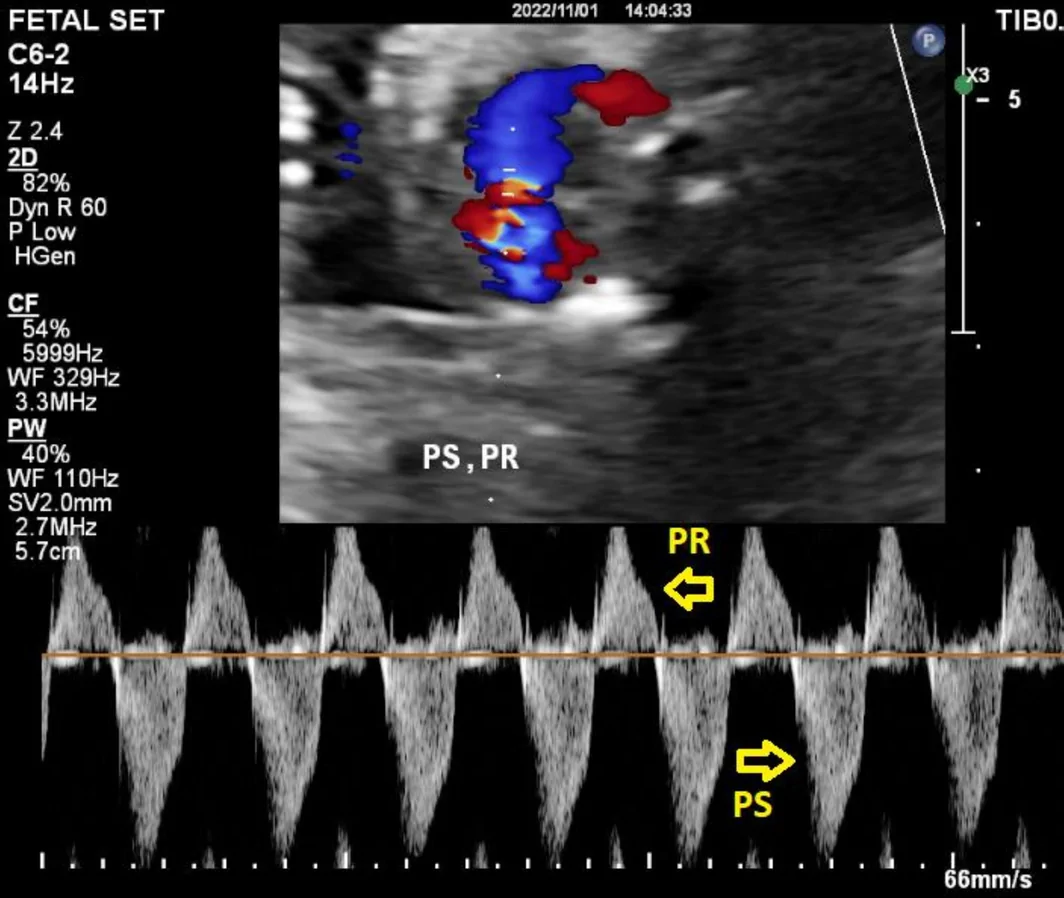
Spectral Doppler tracing of the pulmonary artery demonstrates severe pulmonary stenosis (PS) with prominent regurgitant diastolic flow (PR).
